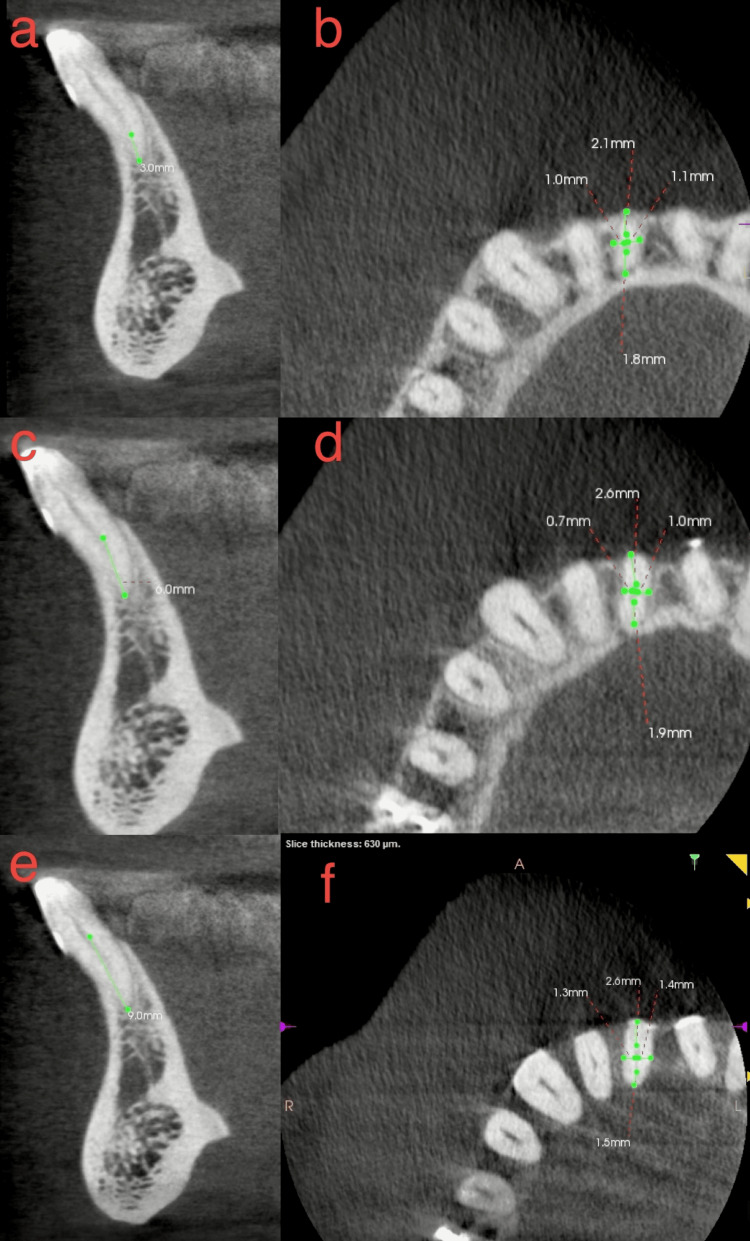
Multiple CBCT depicting measurement of root dentin thickness. (a, c, e) Measurement of root level at 3, 6, 9 mm from the apex, respectively. (b, d, f) Measurement of dentin labially, lingually, distally, and mesially at 3, 6, 9 mm from the apex. CBCT - Cone beam computed tomography
